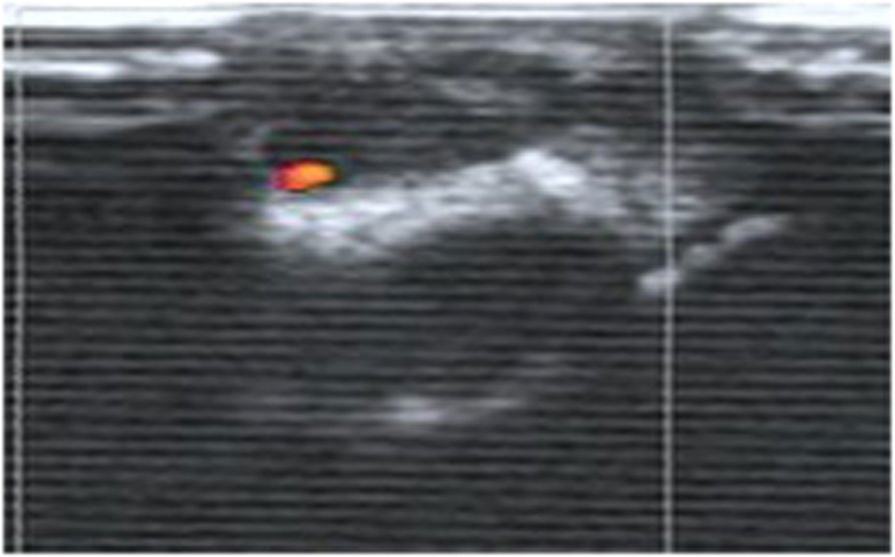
A 24-year-old man with a painless mass on the flexor surface of the index finger. Sonography shows a homogeneous hypoechoic nodule with a small amount of blood flow (Class I)
